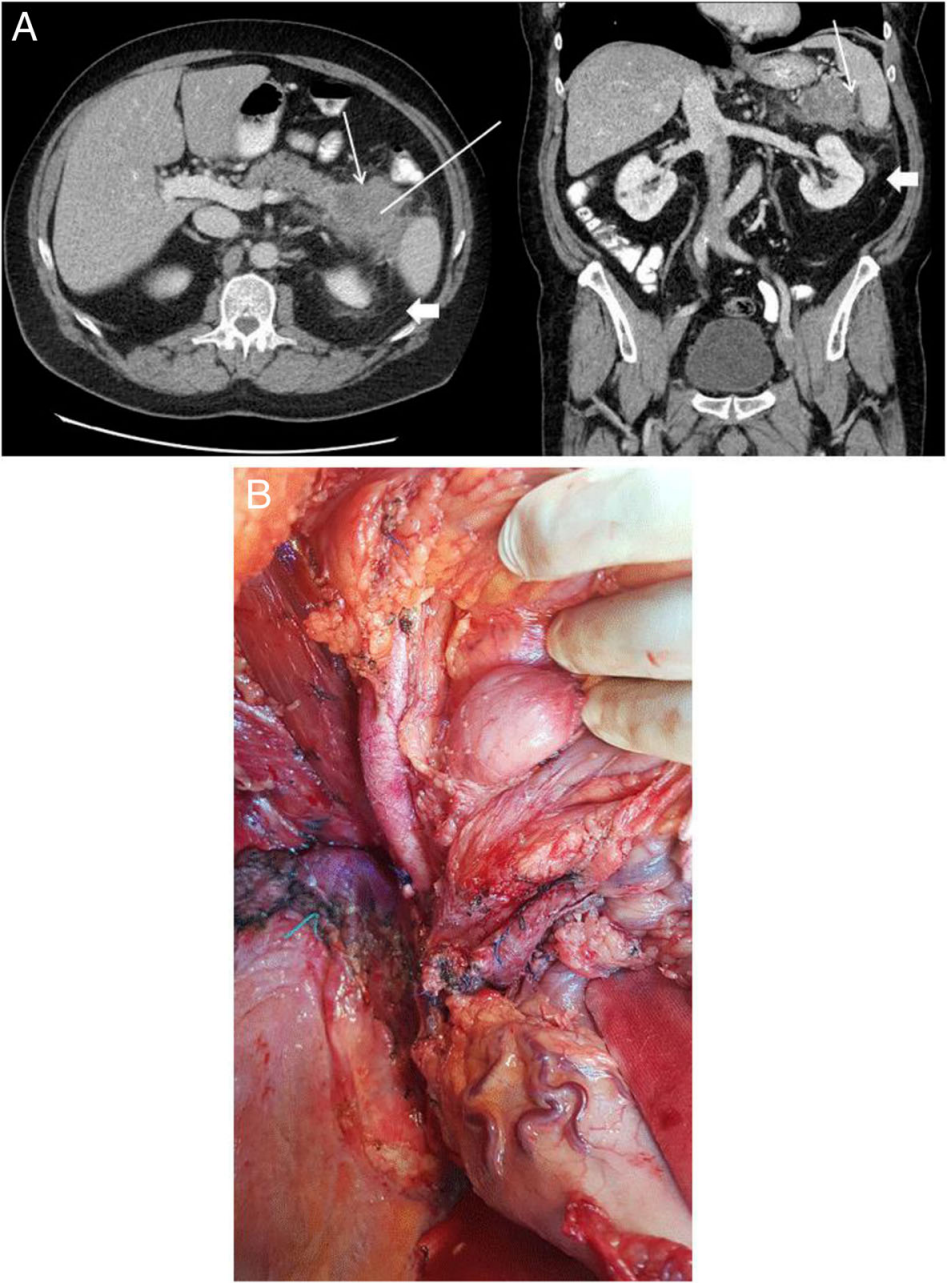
a preoperative CT-scan (left – axial, right – coronal), showing a central hypodense tumor mass of 6,4 × 6,8 cm in the pancreatic tail (marked with white arrow) and a perifocal, diffuse fibrotic enhancement of the retroperitoneal fat (marked with white arrow head). The corridor of the CT core needle biopsy is marked with a white line; b intraoperative situs showing
